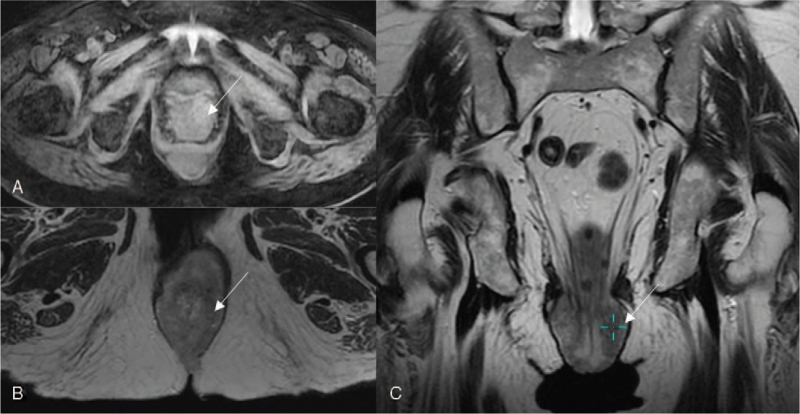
Axial LAVA image after intravenous contrast administration (A) and T2-weighted: axial (B) and coronal (C) images. A lesion measuring 40 × 20 × 25 mm was identified in the cervix, hyper intense in LAVA sequence (A) and of low signal on T2-weighted images (B, C). There is no evidence of vaginal mucosa, bladder and rectal invasion.
